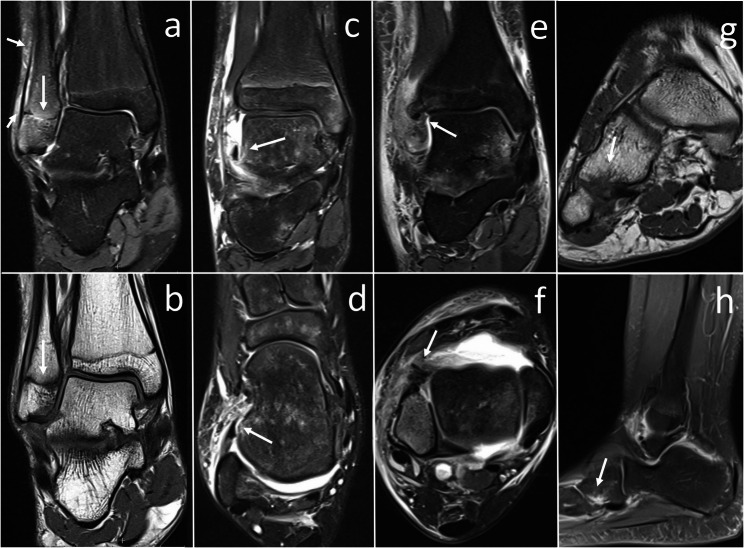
Representative MRI images of common paediatric ankle injuries. (a, b) Coronal IMT TSE images with FS and T1-weighted images in a 14-year-old female patient. Arrows indicate a SH1 fracture accompanied by an associated subperiosteal haematoma. (c, d) Coronal and transverse IMT TSE images with FS in a 10-year-old male patient. Arrows indicate a bony avulsion of the ATFL at its talar attachment, representing an injury of the LCL. (e, f) Coronal and transverse IMT TSE images with FS in a 17-year-old male patient. Arrows mark frayed and displaced fibres of the anterior syndesmosis, consistent with a syndesmotic lesion. (g, h) Coronal T1-weighted and sagittal IMT TSE images with FS in a 13-year-old female patient. Arrows highlight a bone bruise/marrow oedema and an occult impaction fracture of the cuboid. ATFL – anterior talofibular ligament; FS – fat saturation; IMT – intermediate-weighted; LCL – lateral collateral ligament; SH1 – Salter-Harris Type 1; TSE – turbo spin echo
